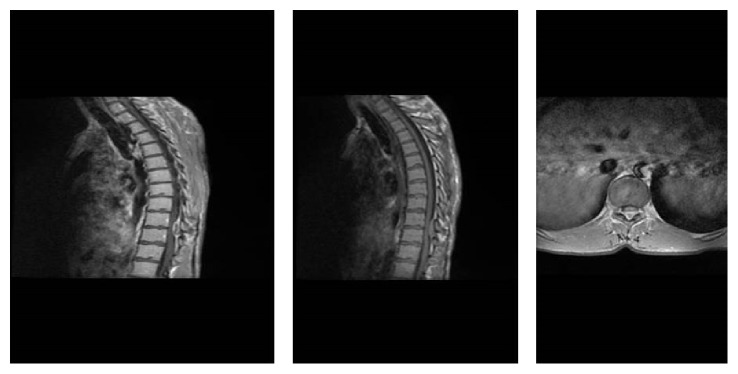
Preoperative thoracic sagittal T1, T2 MRI and axial T2 MRI.
